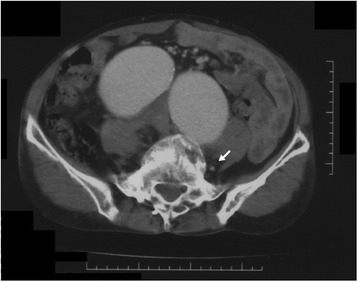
Computed tomography showing bilateral common iliac artery aneurysms and a dilated vena cava. Postoperatively, we could detect the enhanced paravertebral vein (*arrow*). The non-enhanced vena cava indicated an arteriovenous fistula located distal to the vena cava
